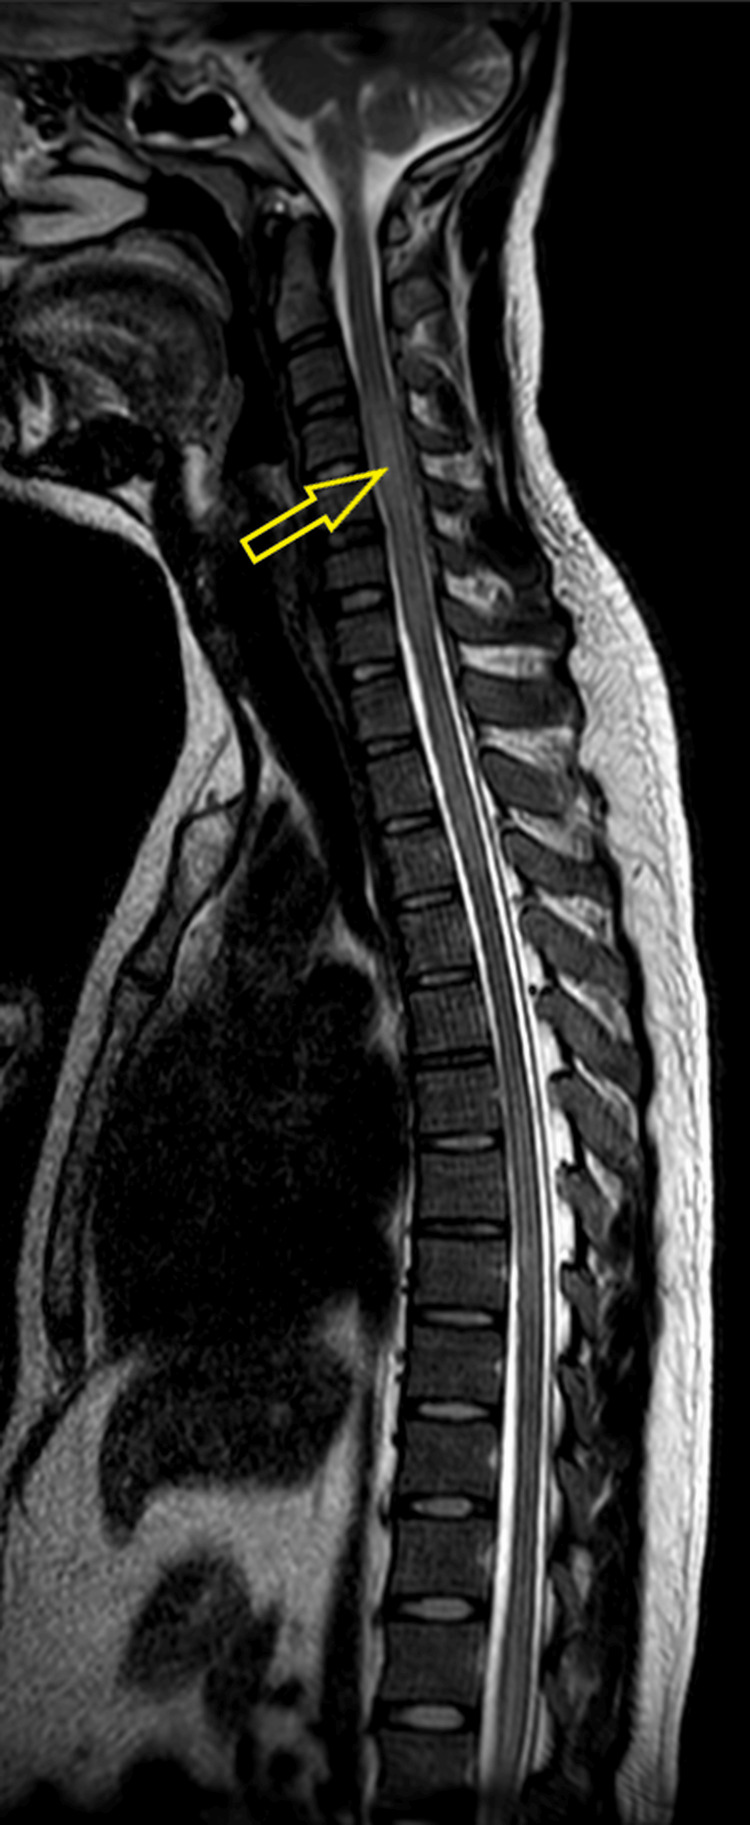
Sagittal T2-weighted MRI of the spine showing intramedullary signal hyperintensity and cord swelling extending from vertebral level C3 to T3, T6 to T9, and T11 to at least L1.
